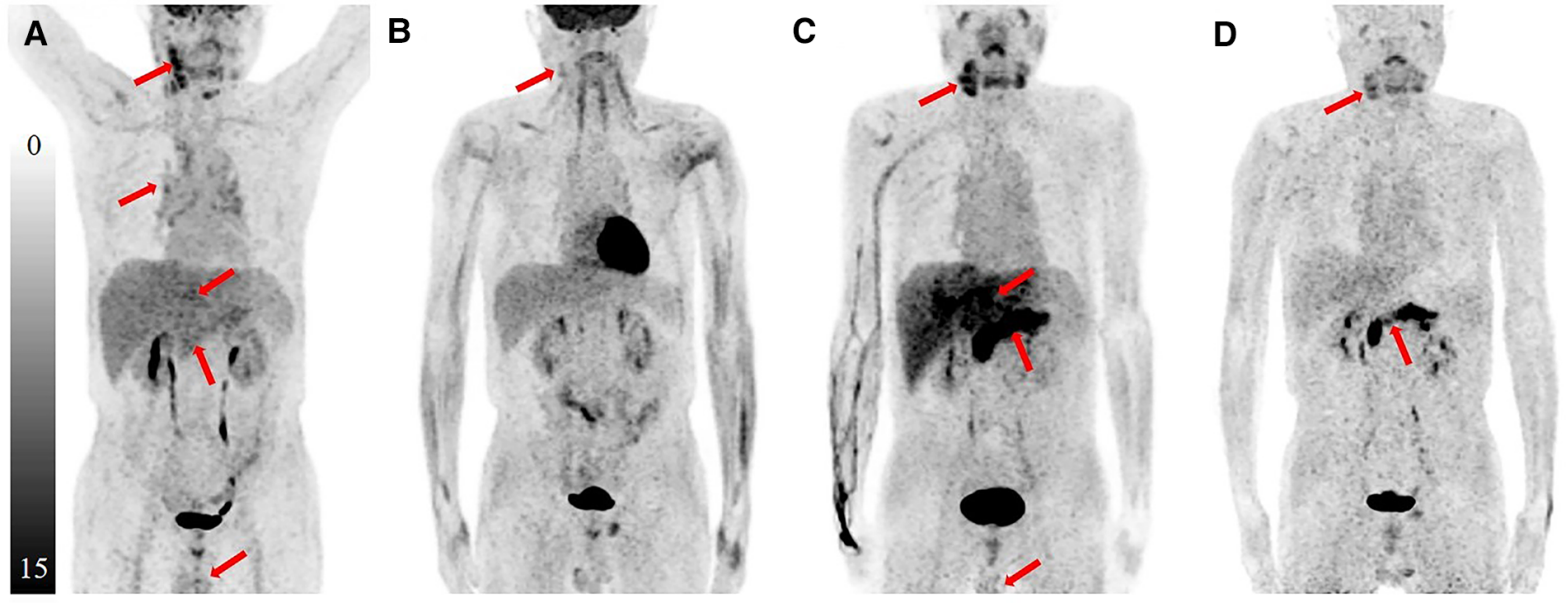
The MIP images of ^18^F-FDG PET/CT and ^68^Ga-FAPI PET/CT. The pre-treatment ^18^F-FDG PET/CT (A) showed elevated uptake of the following lesions: cervical and mediastinal lymph nodes, intrahepatic bile ducts, pancreas, and prostate. The pre-treatment ^68^Ga-FAPI PET/CT (C) indicated more obvious uptake in most lesions mentioned above except for the cervical and mediastinal lymph nodes; besides that, submandibular glands were also involved. After prednisone treatment, FDG-avid lesion was detected only in the right cervical lymph node (B), but the raised uptake of ^68^Ga-FAPI could still be observed in the pancreas and submandibular glands (D).
